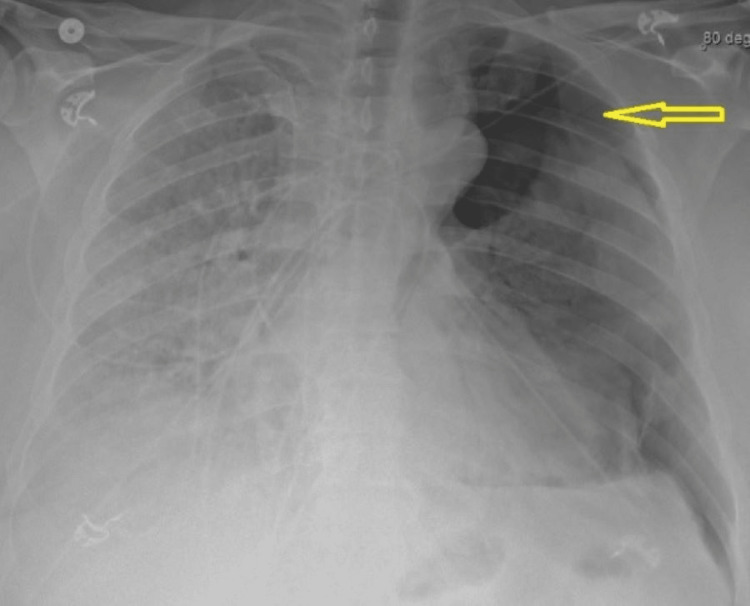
Chest X-ray. Yellow arrow points toward the apical pneumothorax. Considerable opacification of the lung fields was also noted.
